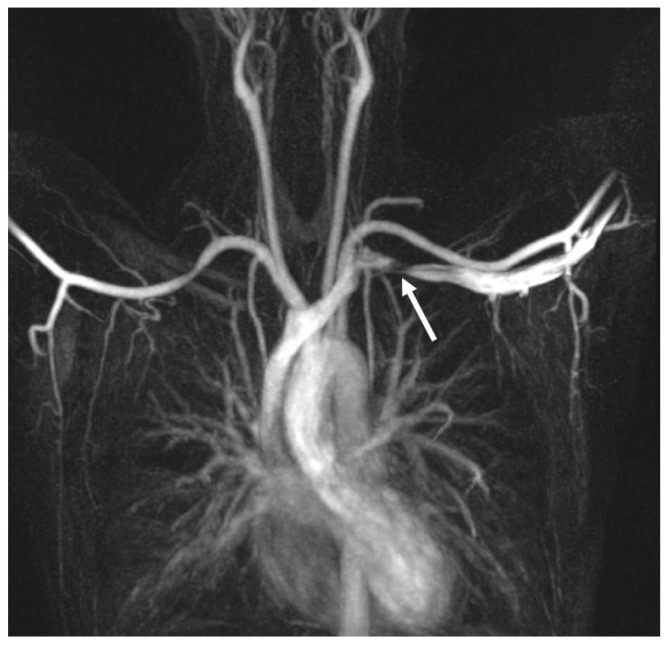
MRI disclosing left subclavian vein stenosis (white arrow) after ipsilateral arm abduction.
